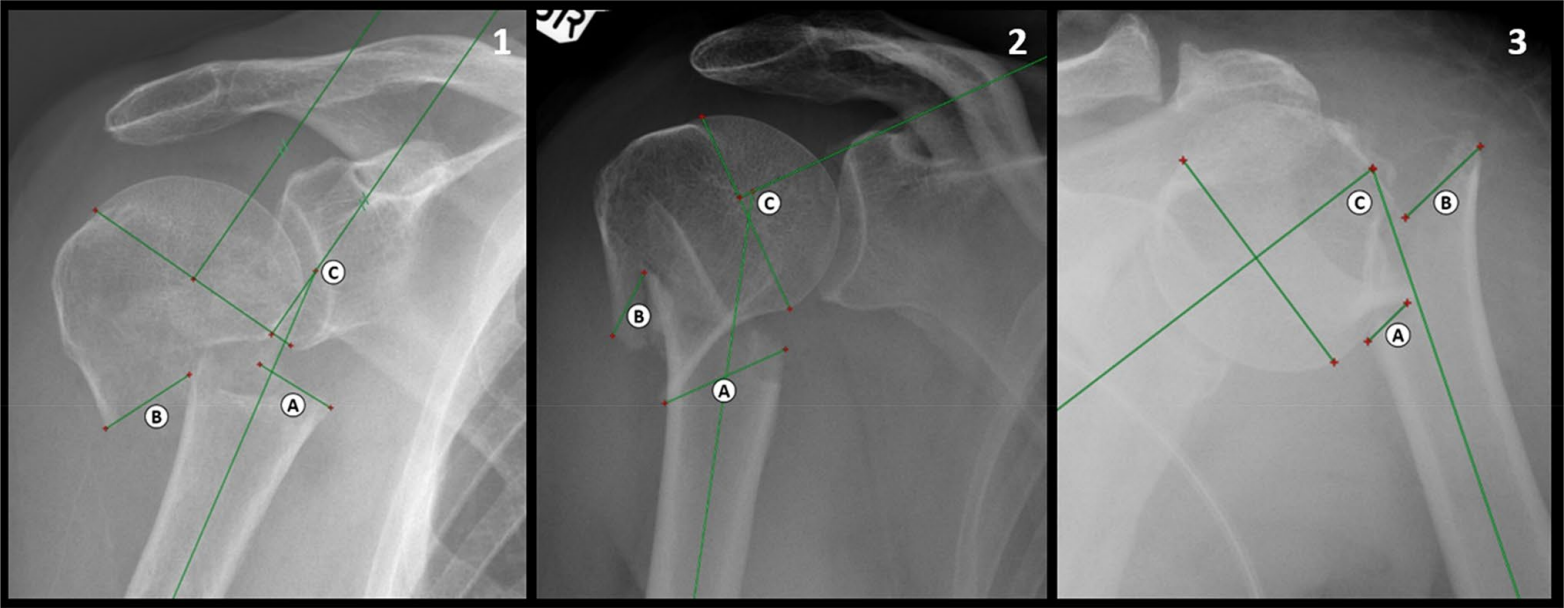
1 = type A (partial medial shaft displacement with valgus angulation), 2 = type B (entire medial and ventral shaft displacement without humeral head tilt), 3 = type C (lateral shaft displacement with varus angulation). Three parameters were measured on each radiograph: A = maximal medial gap, B = maximal lateral gap, C = neck-shaft angle
